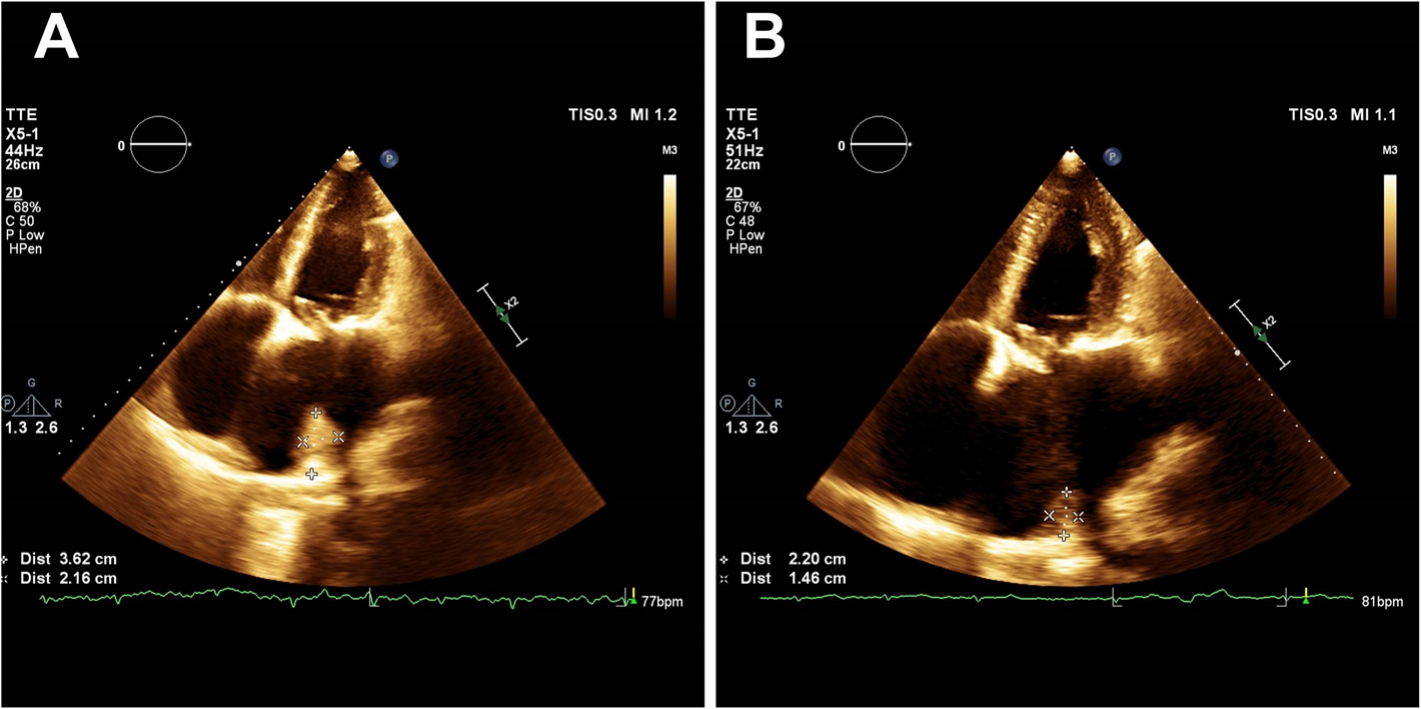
Echocardiograms 18 and 21 months post-surgery The apical four-chamber views showing a partially resolved thrombus attached to the posterior wall of the left atrium during the visits at 18 months (A) and 21 months (B) postsurgery
